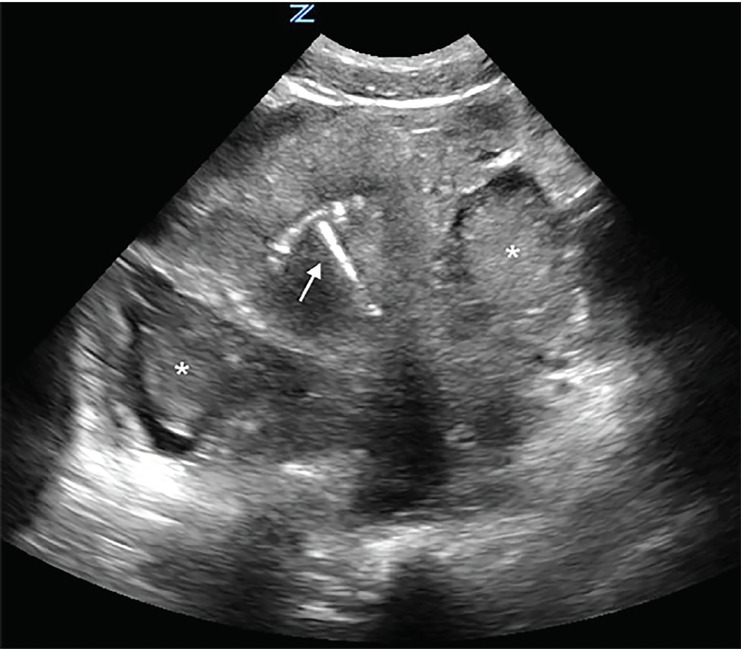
Transabdominal transverse ultrasound view of the pelvis. Note presence of intrauterine device within uterus (arrow) and pelvic hematoma (asterisks).
